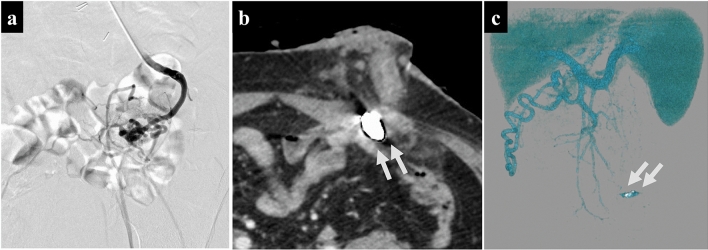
Percutaneous transhepatic obliteration. a Fluoroscopic angiography revealed that the peristomal varices were visualized from the inferior abdominal wall vein to the external iliac vein. b, c Postoperative contrast-enhanced CT revealed the disappearance of stomal varices (arrow) (b axial, c 3D angiography)
